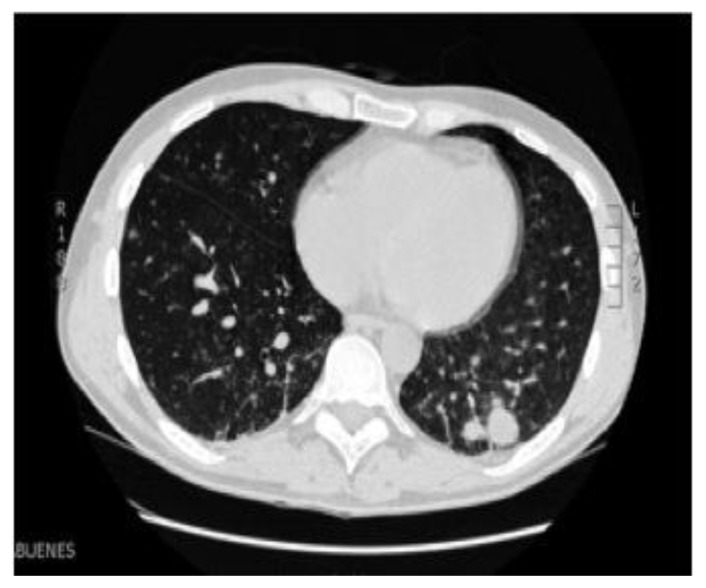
Computed tomography show pulmonary nodular lesions in middle and lower lobes.
